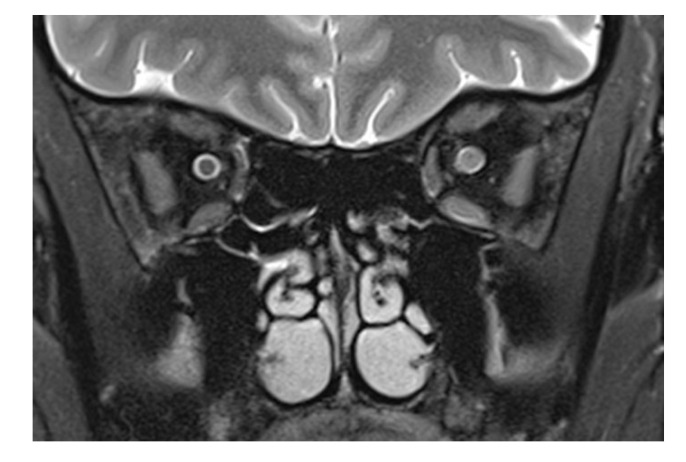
T2-weighted frontal image in a multiple sclerosis patient with optic neuritis. While the subarachnoid space has a normal diameter on the left, an enlarged optic nerve encroaches in the same space, recognizable by the smaller liquor-filled rim of the nerve. Leakage of cerebrospinal liquor after direct laceration of the sheath, however, cannot be counted out. The diffuse signal increase of the nasal retrobulbar space in Figure 1f,g may be explained by cerebrospinal liquor leaking from an injured optic nerve sheath and by edema.
